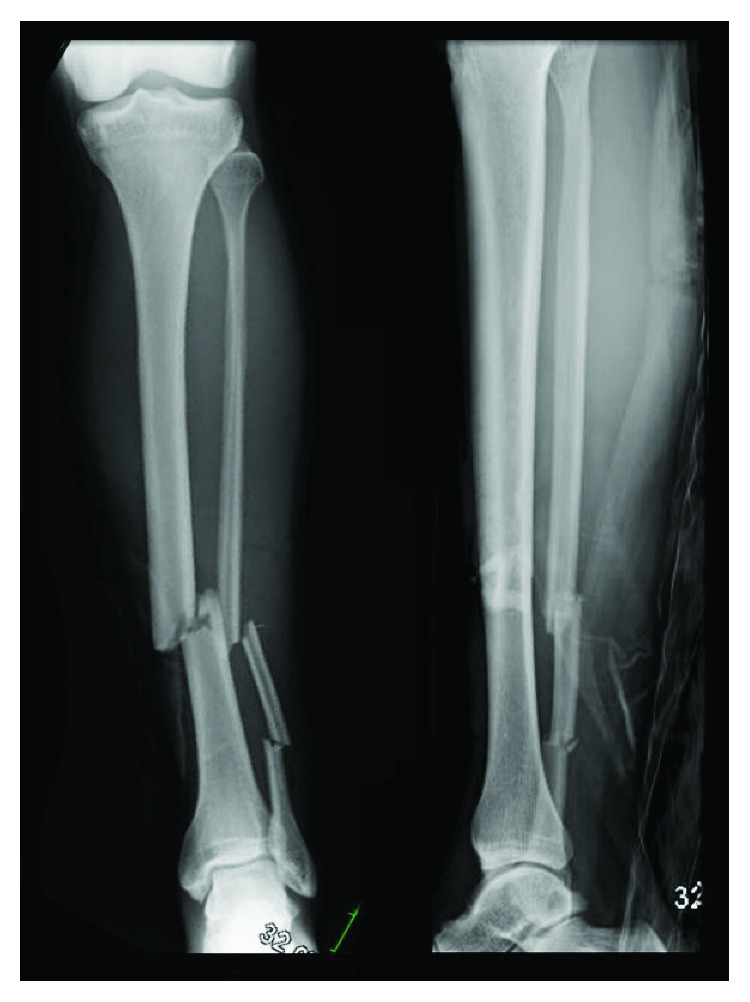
Anteroposterior (AP) and lateral radiographs of the left leg demonstrating a transverse distal diaphyseal tibial fracture and segmental fibular fracture.
